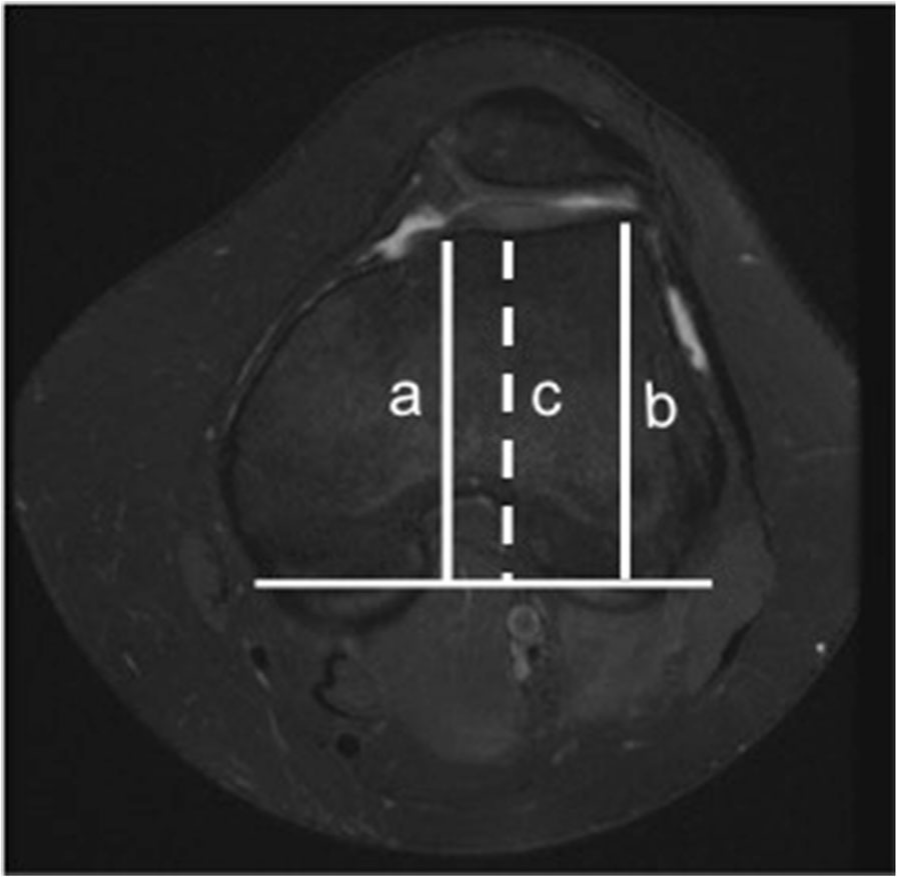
Trochlear depth assessment. Axial PDFS left knee MR image, demonstrating the method used for the measurement of trochlear depth. First, a line is drawn paralleling the posterior femoral condyles surfaces. Perpendicular to this baseline, trochlear depth is calculated by measuring the mean of the maximum AP distance of the medial (a) and lateral (b) femoral condyles minus the distance between the deepest point of the trochlear groove and the line paralleling the posterior condylar surfaces (c). Trochlear depth = [(a + b)/2] − c
